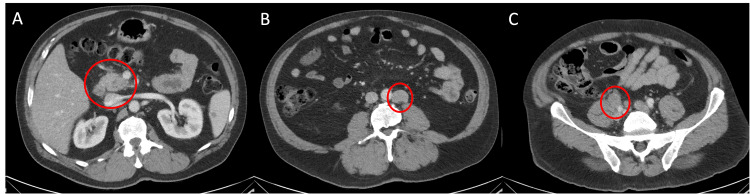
CT of abdomen and pelvis Axial view of the abdomen demonstrates haziness of the pancreatic head concerning for pancreatitis in image (A). Image (B) identifies the 2.7 x 2.0 cm enlarged left para-aortic lymph node. Image (C) identifies the enlarged right common iliac node, measuring 2.6 x 2.2 cm. CT: Computed tomography
